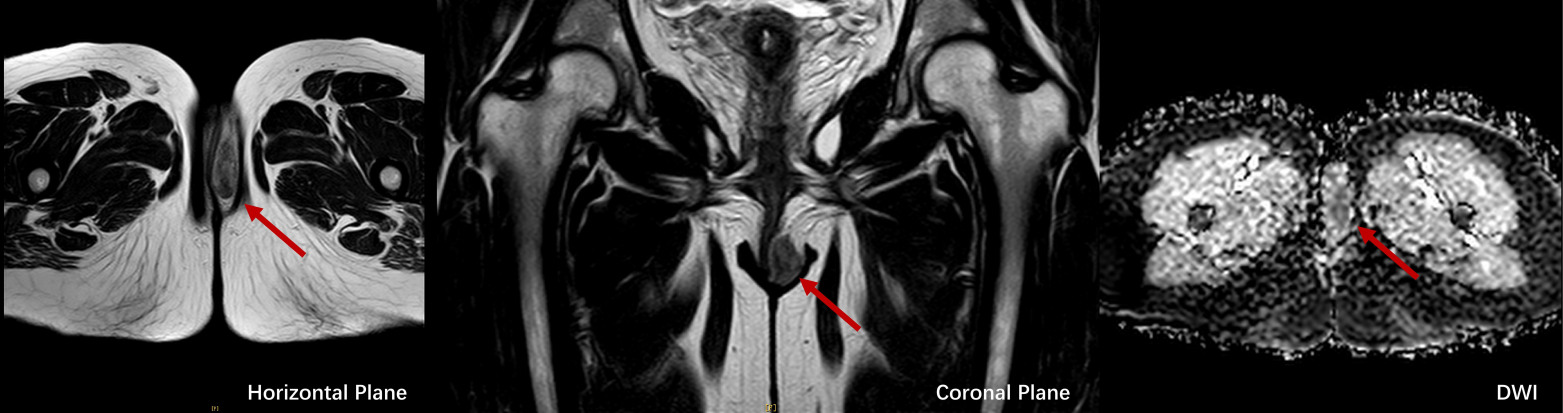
MRI images of LGMFS, indicated by red arrows, in horizontal (left), coronal (middle), and diffusion-weighted imaging (DWI, right) planes, showcasing restricted diffusion and well-defined tumor margins.
